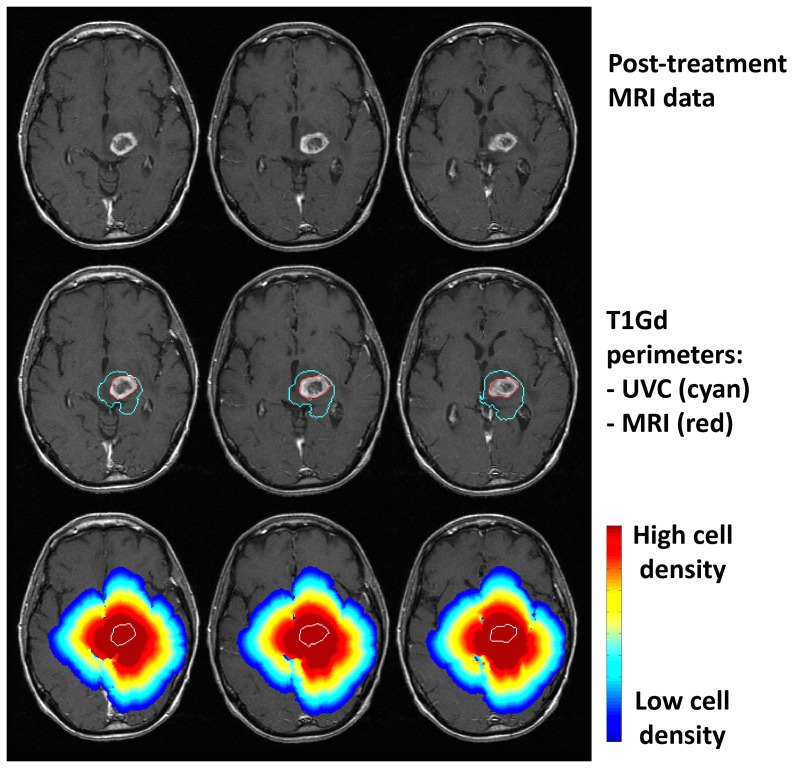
Comparisons between T1Gd MRI data and untreated virtual control (UVC) prediction at post-treatment time point. Patient was 58 years old and underwent biopsy followed by conformal radiation therapy with concurrent temozolomide chemotherapy. Top row: MRI data. Middle row: Actual tumor perimeter (red) with superimposed UVC-predicted tumor perimeter (cyan). Bottom row: full distribution of UVC cell densities showing invasion peripheral to abnormality. Outermost blue cell density profile represents a very low, but non-zero, threshold. Perimeter of actual tumor outlined in white.
